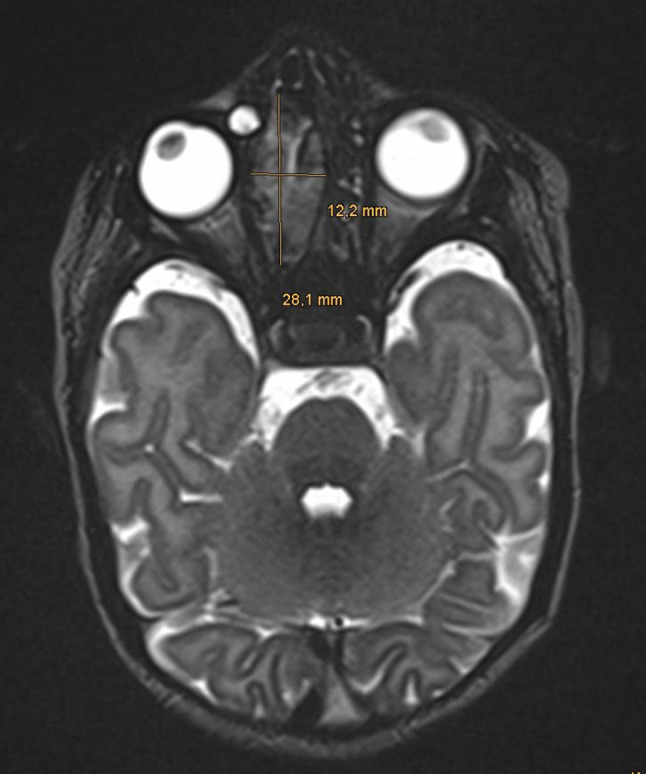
Expansion of Kaposiform Haemangioendothelioma in axial plane in T2 weighted MRI scan, note the compression of the right tear duct
